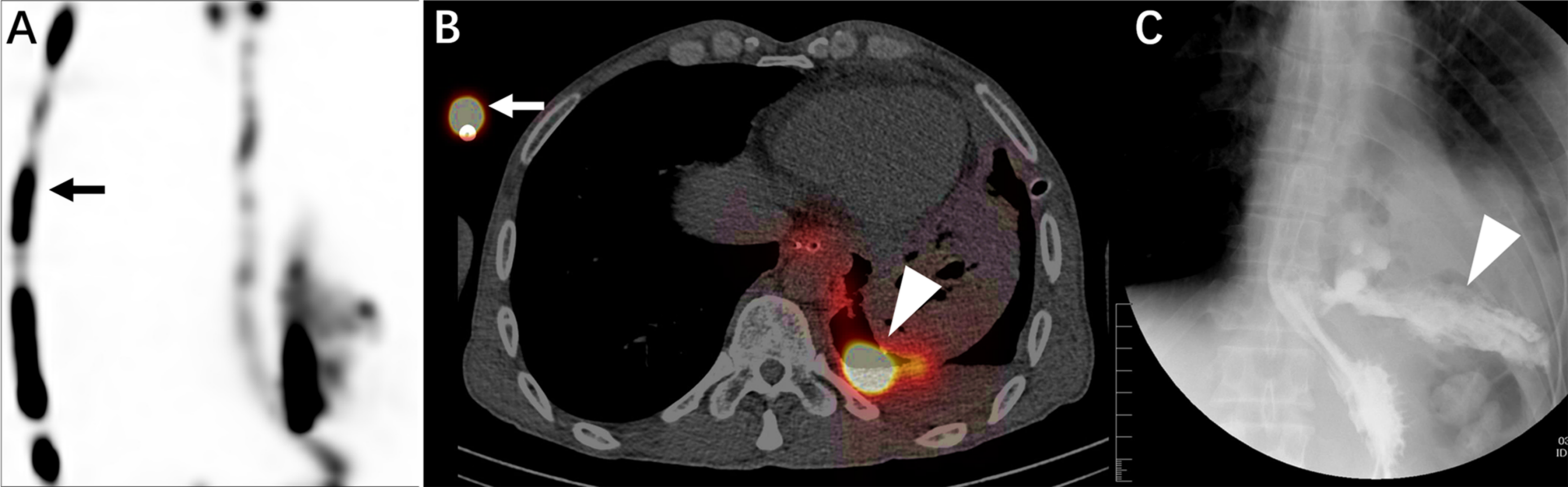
This is a 48-year-old male patient with an oesophageal tumour and requirement for a gastric tube due to inability to eat. The patient underwent salivagram without gastric tube removal. Whole SPECT imaging (A), flued SPECT/CT imaging (B), and VFSS imaging (C) are demonstrated. The gastric tube (A, B, straight arrow) is visible. An abnormal concentration of imaging agent is present in the left thoracic cavity (B, C, triangular arrow).
